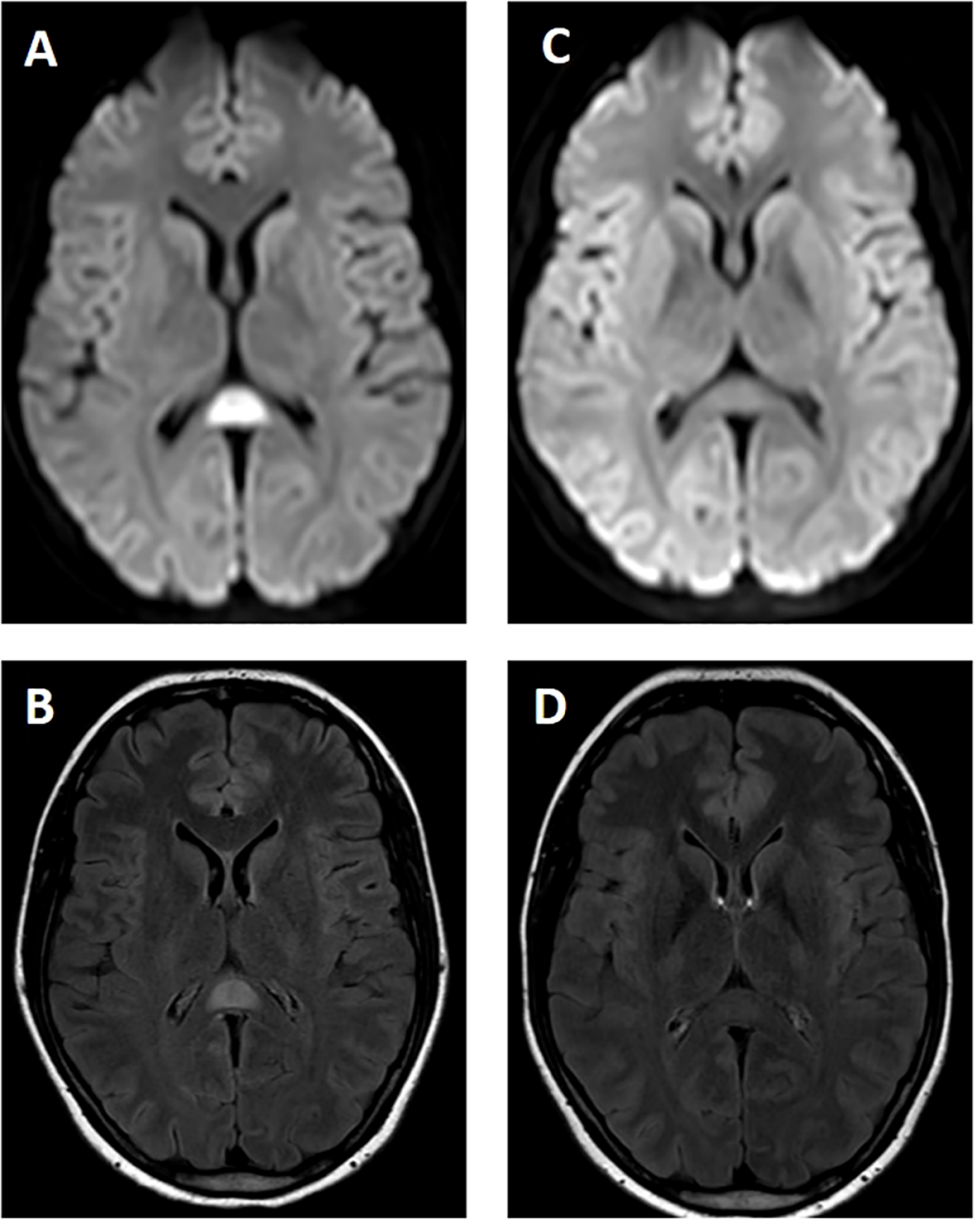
(A, B) MRI of the brain on day 3. A single, ovoid-shaped lesion is seen in the splenium of the corpus callosum with increased signal on diffusion-weighted imaging (A) and fluid inversion recovery sequences (B). (C, D) MRI of the brain on day 22 shows full remission of the lesion in the splenium corpus callosum.
